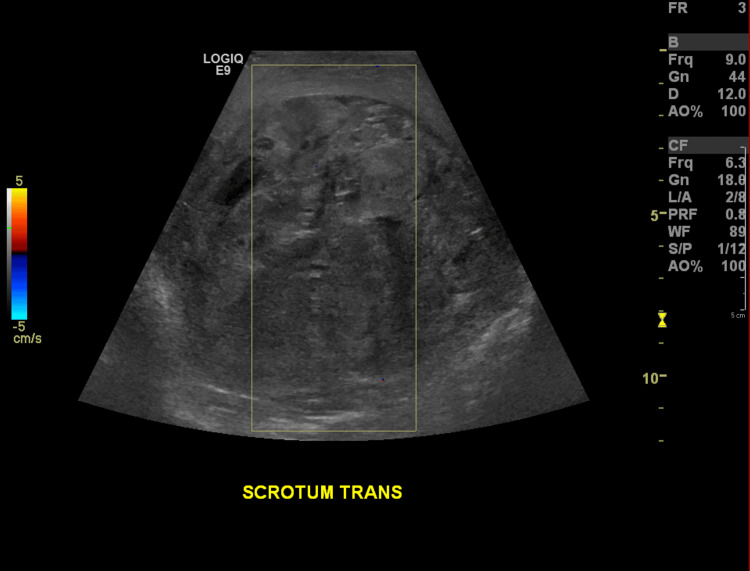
Sonographic image of the right testicle status post-orchidectomy demonstrating a large heterogenous collection within the surgical bed
